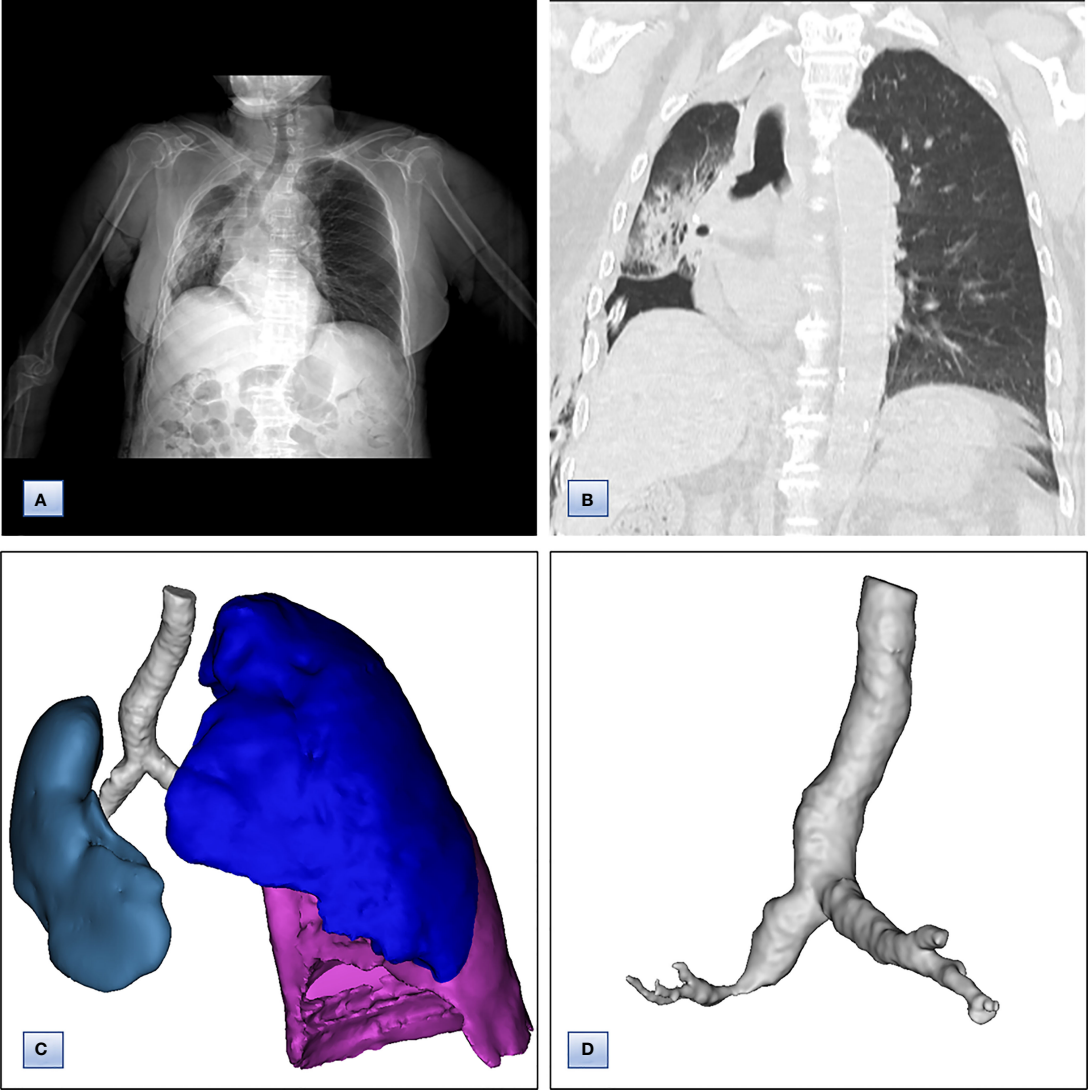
Chest computed tomography and7 3D CT reconstruction after surgery. (A) Chest CT, revealing that the trachea and mediastinum were more obviously shifted to right than that before surgery. (B) Coronal chest CT images, indicating adequate recruitment of the right middle lobe. (C, D) 3D reconstruction of CT images, showing (C) only middle lobe in right thoracic cavity and (D) unobstructed bronchus of right middle lobe.
